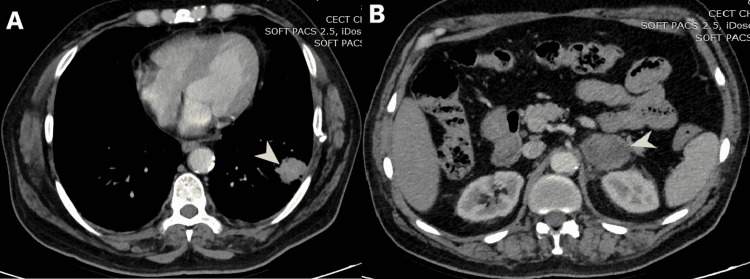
(A) CT scan of thorax, (B) Upper abdomen section of the same scan A: Mediastinal window of contrast-enhanced CT of the thorax showing 3 cm x 2.5 cm heterogeneously enhancing mass-like lesion (arrowhead) in the lower lobe of left lung reaching up to the pleura. B: Upper abdomen sections of the same scan show bulky heterogeneously enhancing left adrenal gland (arrowhead) measuring 6 cm x 4.5 cm.
